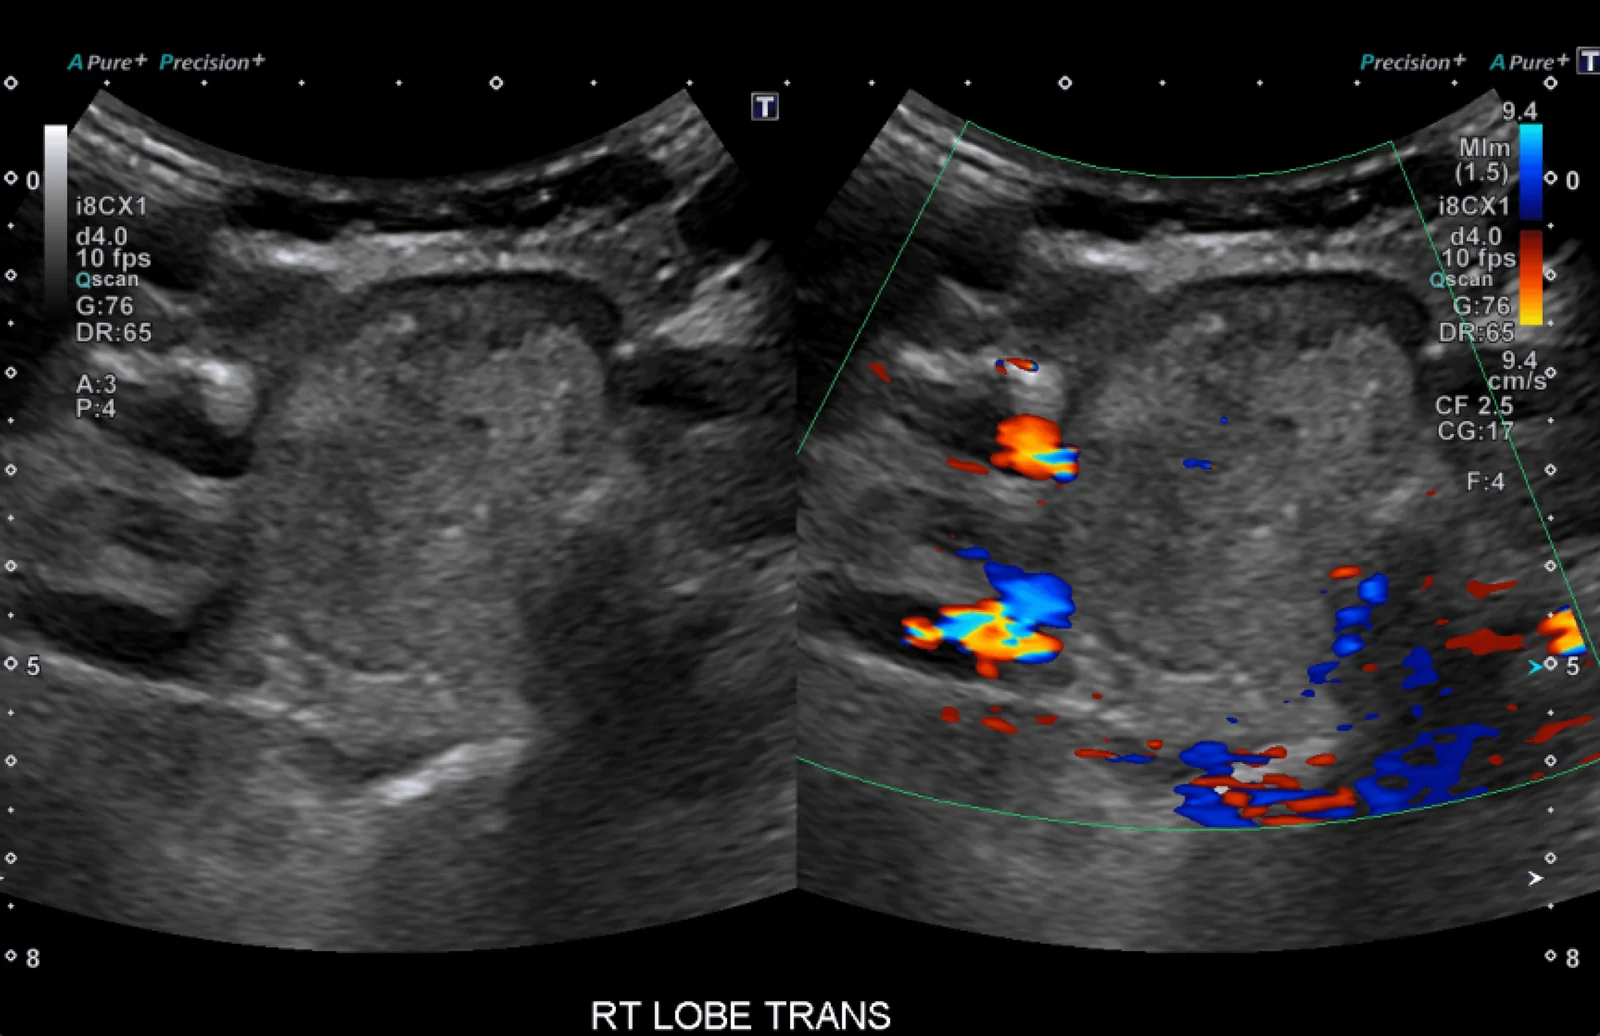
Ultrasound of the thyroid gland demonstrating the right thyroid lobe with an enlarged nodule
